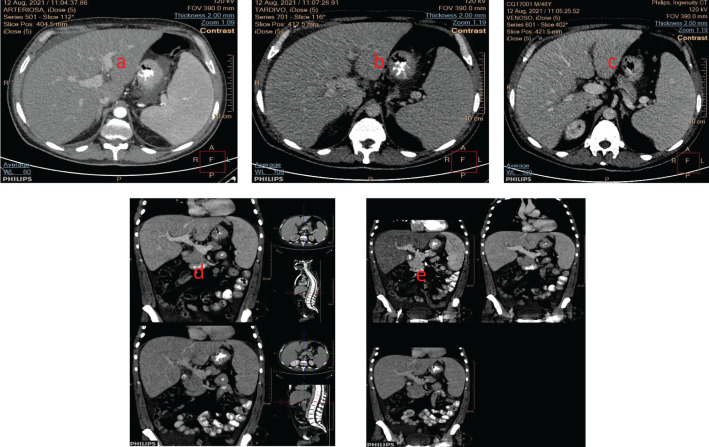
Hepatosplenomegaly was seen in the CT-scan (coronal section) in the (a) arterial phase; (b) venous phase, and (c) portal phase (d) portal (up), venous (down) phases; (e) arterial (left), portal (right) and venous (down) phases (sagittal sections).
